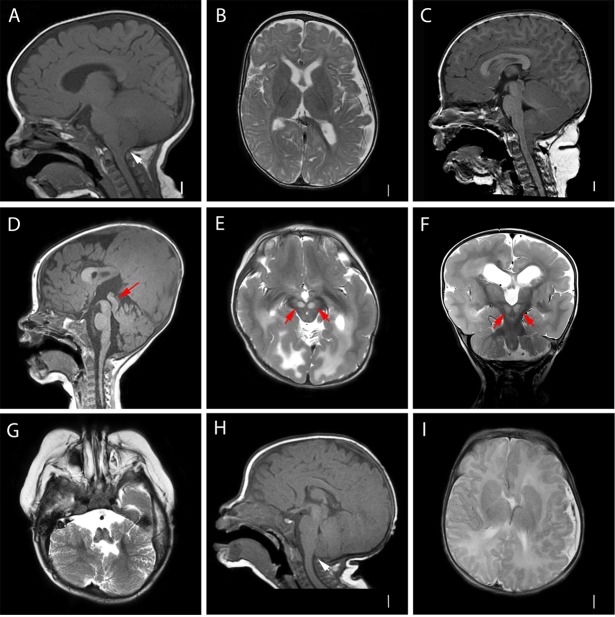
Brain MRI images of *PIK3CA* mutation–positive patients. (A and B) LR11-082. T1-weighted mid-sagittal (A) and T2-weighted axial (B) image at age 6 months showing a large cerebellum, crowded posterior fossa with cerebellar tonsillar ectopia, and a relatively normal cortical gyral pattern (arrowhead). (C) LR12-184. T1-weighted mid-sagittal image showing marked cerebellar tonsillar ectopia (arrowhead). (D–H) LR12-183. T1-weighted mid-sagittal (D), T2-weighted axial (E and G), and coronal (F) images showing diffuse cortical dysplasia, partial agenesis of the corpus callosum, dysplasia/hypoplasia of the cerebellar vermis and hemispheres, diffuse dysmyelination, abnormal high T2 signal intensities in the red nuclei and thalami bilaterally (red arrows in E and F), and a very large tectum (red arrow in D). (H and I) LR13-197. T1-weighted mid-sagittal (H) and T2-weighted axial (I) images showing bilateral cortical dysplasia, hippocampal dysplasia, white matter dysmyelination, and bilaterally dysplastic ventricles. There is mild cerebellar tonsillar ectopia (arrow in H).
